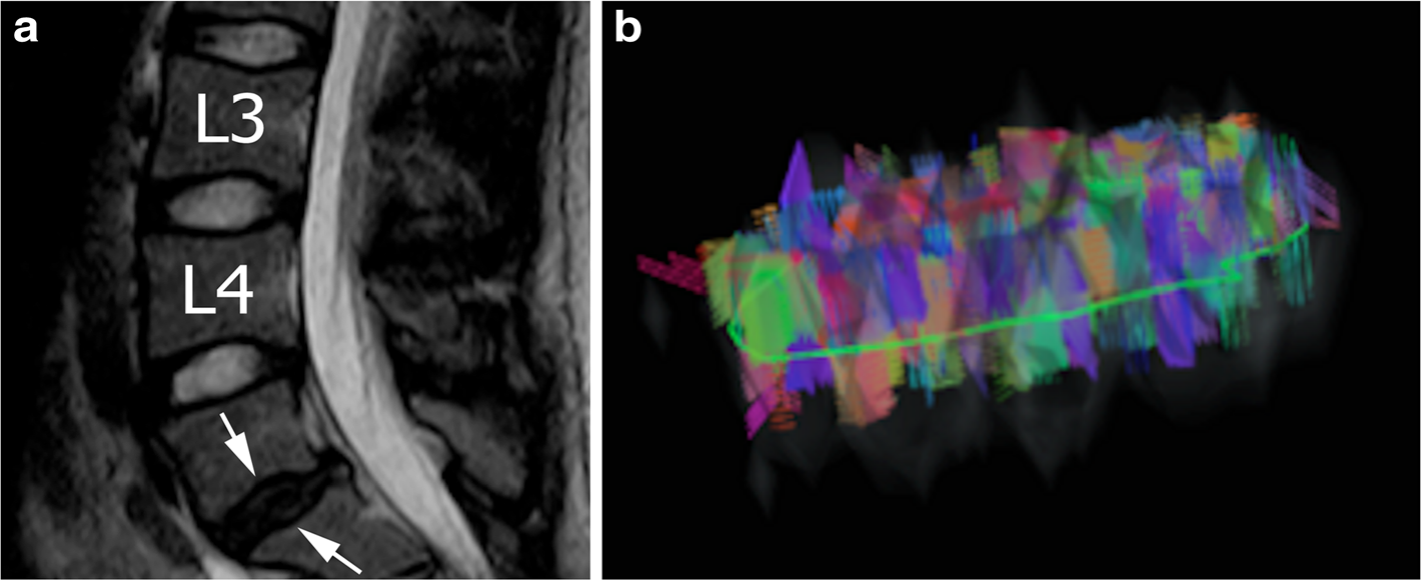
Magnetic resonance of the lumbar spine performed on a of 31-year-old man. a Sagittal T2-weighted image shows protrusion of a thinned and dehydrated intervertebral disc (Pfirrmann IV, *arrows*) at L5-S1. b Tractograpy shows irregular, disordered and thin fibres
